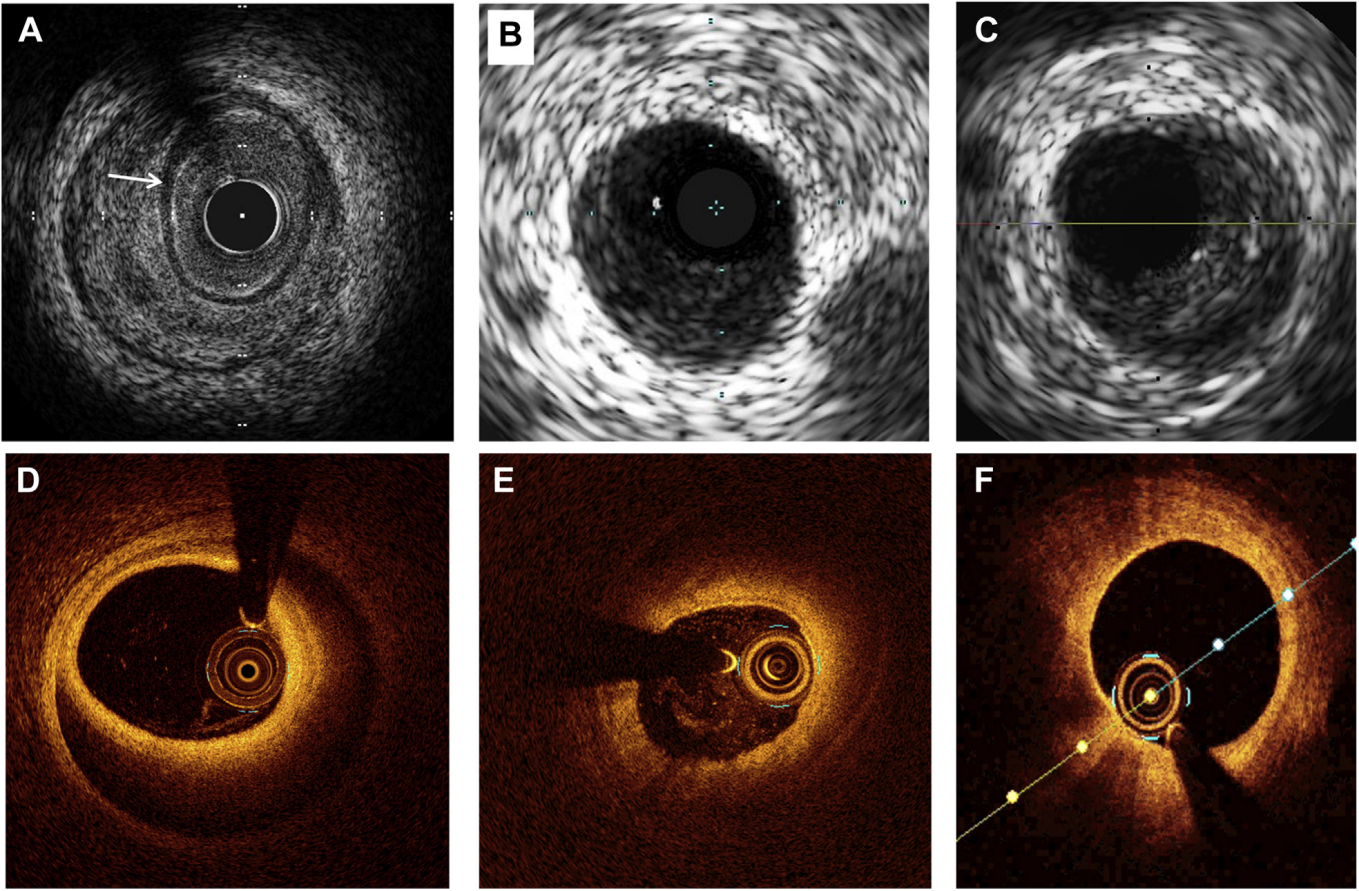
IVUS and OCT Images Intravascular ultrasound (IVUS) image of spontaneous coronary artery dissection (SCAD) (A) showing classical white-black-white appearance of the intimal-medial membrane, which is pathognomonic of SCAD (arrow). When not present, SCAD (B) can be very difficult to distinguish from lipidemic atherosclerotic plaque (C) on IVUS. Careful scrutiny of the entire pull back length may be required in such cases. Optical coherence tomographic (OCT) imaging of SCAD (D) clearly showing the intimal-medial membrane, false lumen, and external elastic lamina. Sometimes light penetration to the external elastic lamina may be limited (E), but with careful inspection OCT imaging can usually be distinguished from lipidemic atherosclerotic plaque (F).
